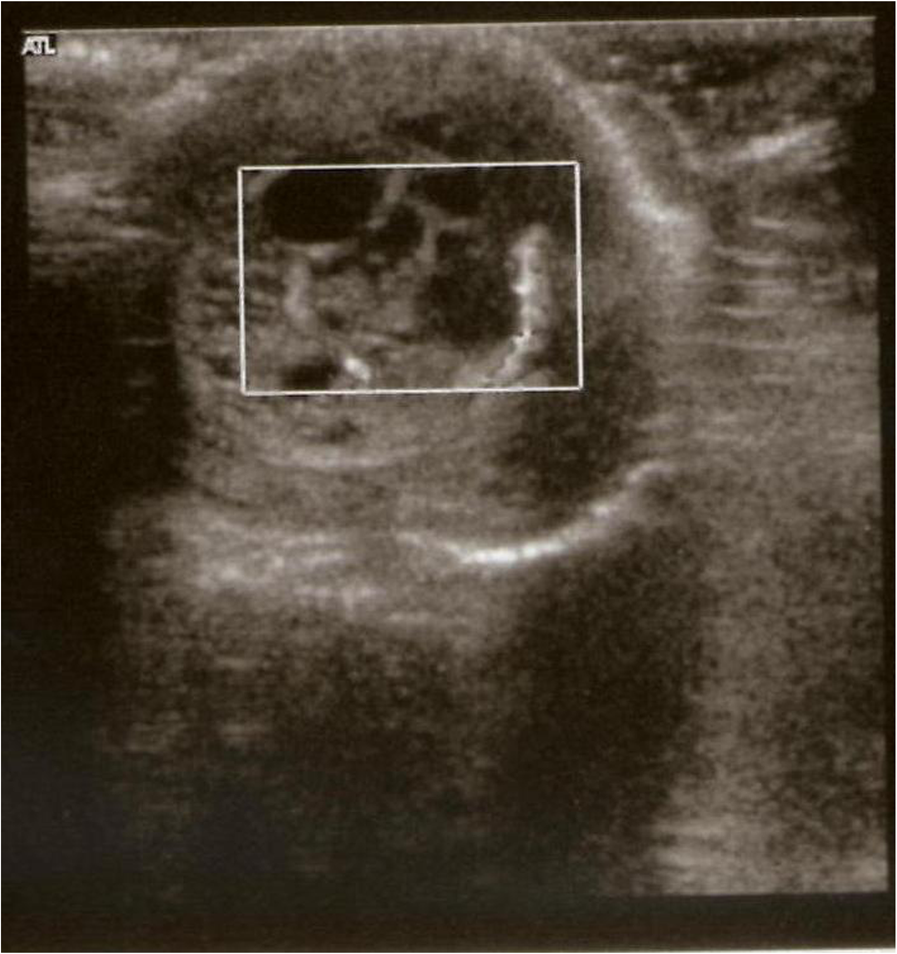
Ultrasound image obtained at birth revealing enlarged left testicle (1.57 × 1.33 cm) and a well encapsulated, complex cystic mass.
